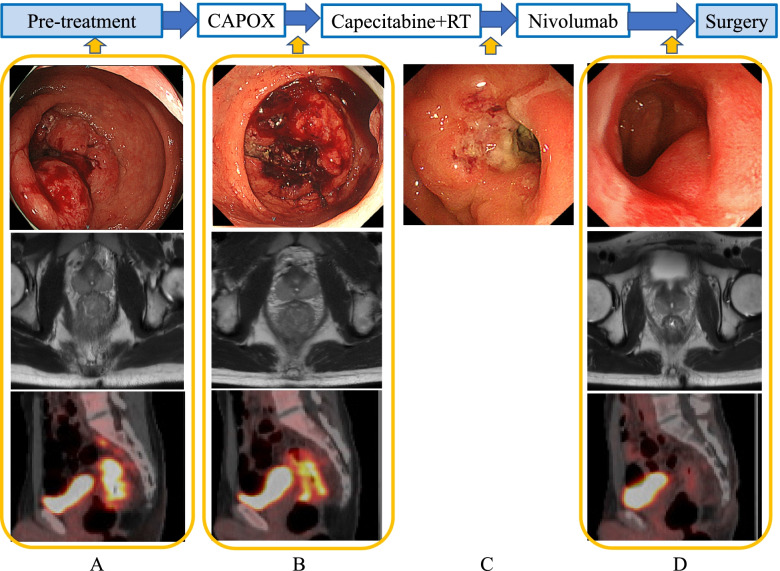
Clinical imaging in the course of treatment. After administration of CAPOX, the tumor did not shrink, but it started to shrink after capecitabine and radiotherapy. After the administration of nivolumab, the large tumor almost disappeared on imaging. A Colonoscopy, axial MRI, and sagittal PET images before treatment. B Colonoscopy, axial MRI, and sagittal PET images after CAPOX administration. C Colonoscopy images after capecitabine administration and radiotherapy. D Colonoscopy, axial MRI, and sagittal PET images after nivolumab administration
